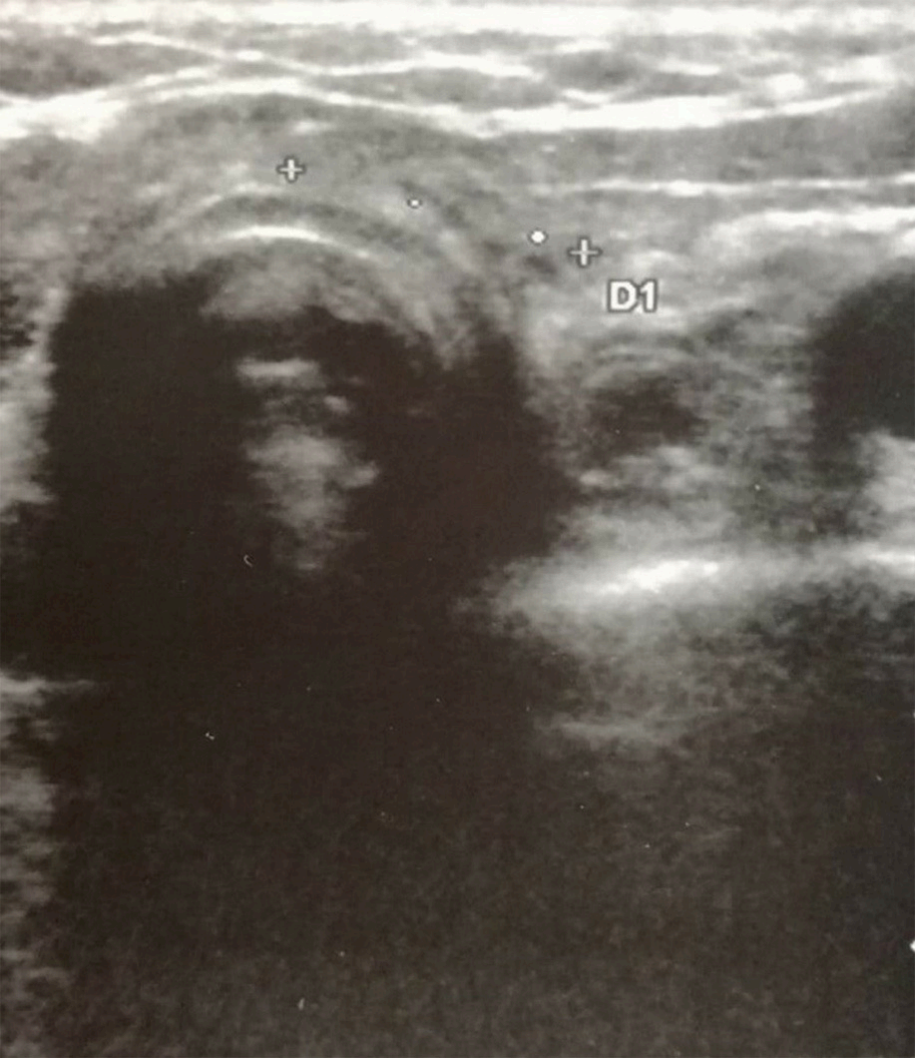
Neck ultrasonography performed at 10-month follow-up visit after the second cycle of intravenous methylprednisolone treatment shows complete regression of the two hypoechoic areas in the thyroid bed.
